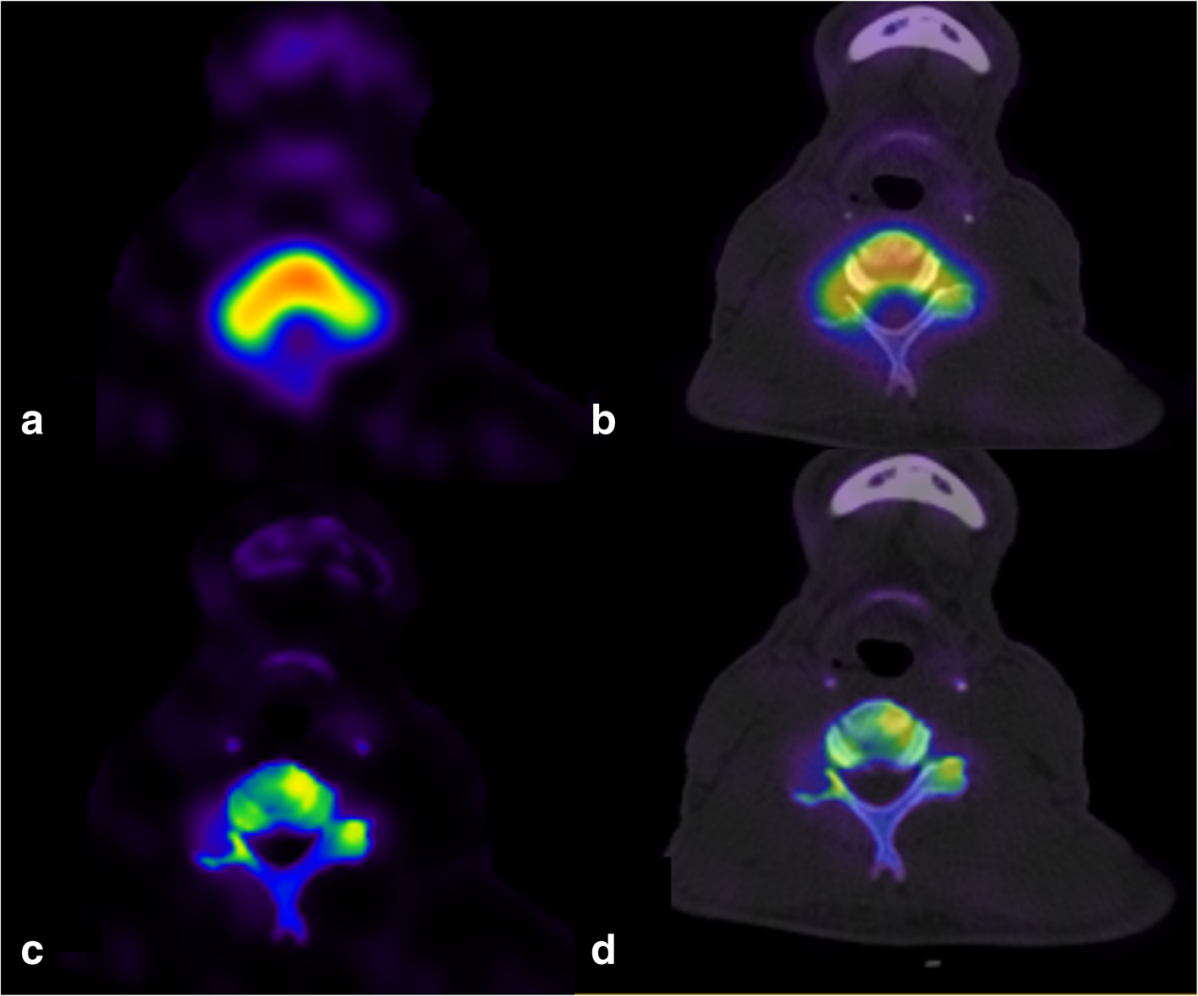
Patient presented with neck pain for evaluation. Detailed Legend: Subsequent xSPECT and SPECT fusion at the C6 level are shown. a SPECT, b SPECT/CT, c xSPECT, and d xSPECT/CT. Final diagnosis was metastatic adenocarcinoma of the lung
